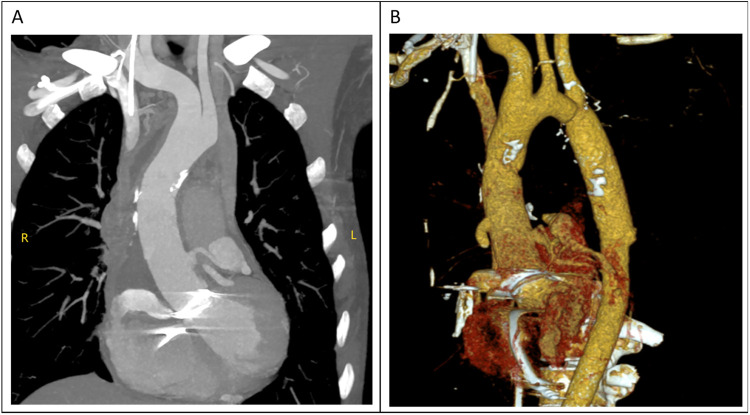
(A,B) the diameter of the ascending aorta had returned to normal in the computed tomography (CT) examination performed after surgery.
